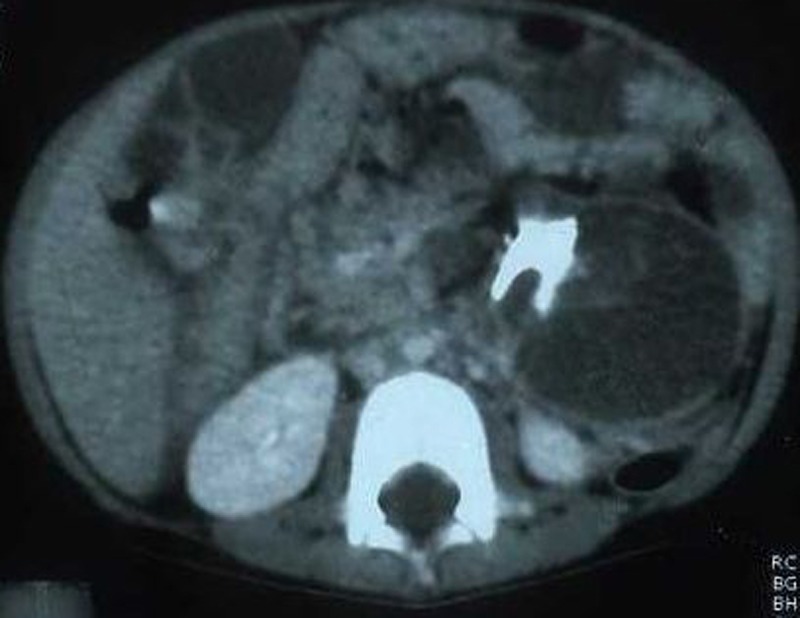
Figure 1: CT scan showing a large heterogenous soft tissue mass showing areas of fat, fluid, soft tissue attenuation with calcifications.
